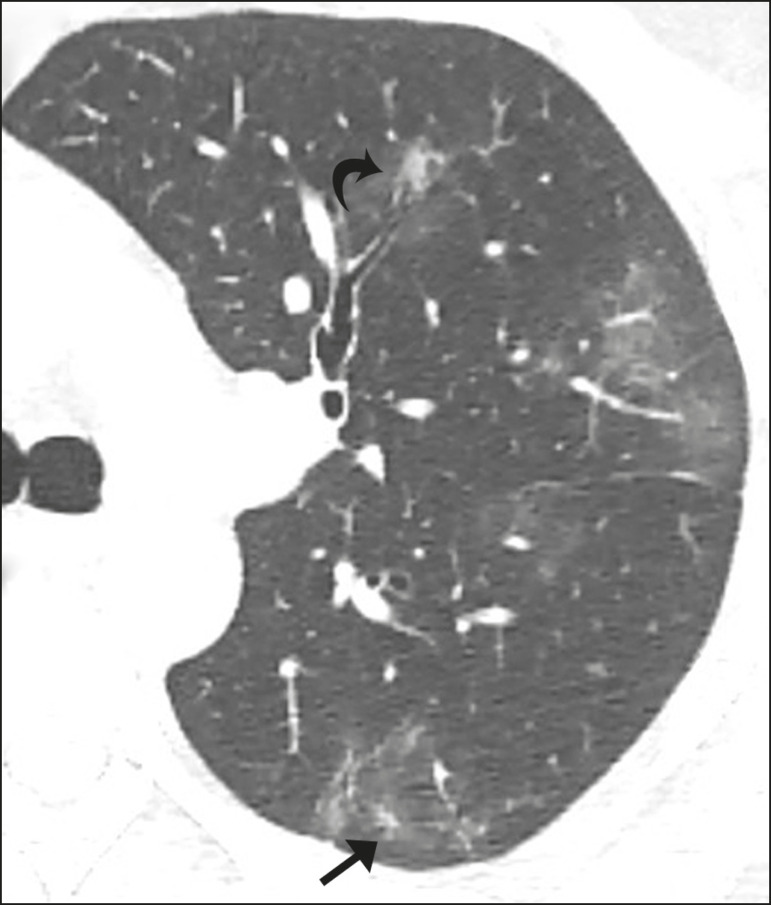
Cross-sectional nonenhanced chest CT image of the left lung in a 38-year-old man (husband of patient showed in Figure 1) with COVID-19 pneumonia shows poorly defined ring-like opacity surrounding a subtle area of ground-glass attenuation and in its center a poorly defined nodular ground-glass opacity surrounding a vascular structure (arrow), resulting in a target sign. Also noted are patchy ground-glass opacities and small poorly defined nodule with halo of ground glass opacity in the left upper lobe (curved arrow).
